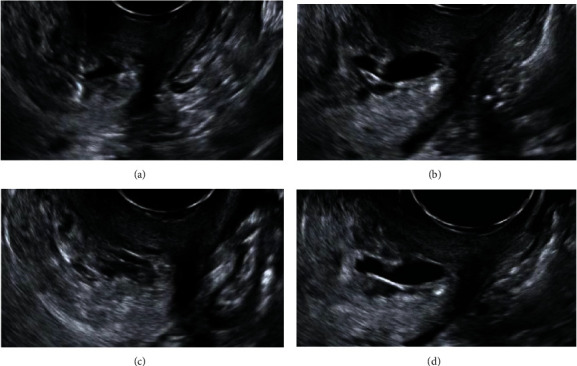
Transabdominal puncture using a 17-gauge needle under transvaginal ultrasound guidance. Aspiration of (a, b) gestational content followed by (c, d) local methotrexate administration.
